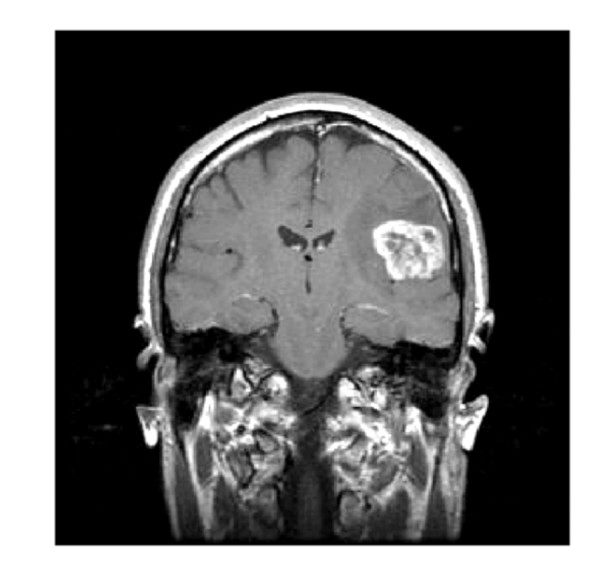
A representative magnetic resonance image of a GBM patient at initial diagnosis.
